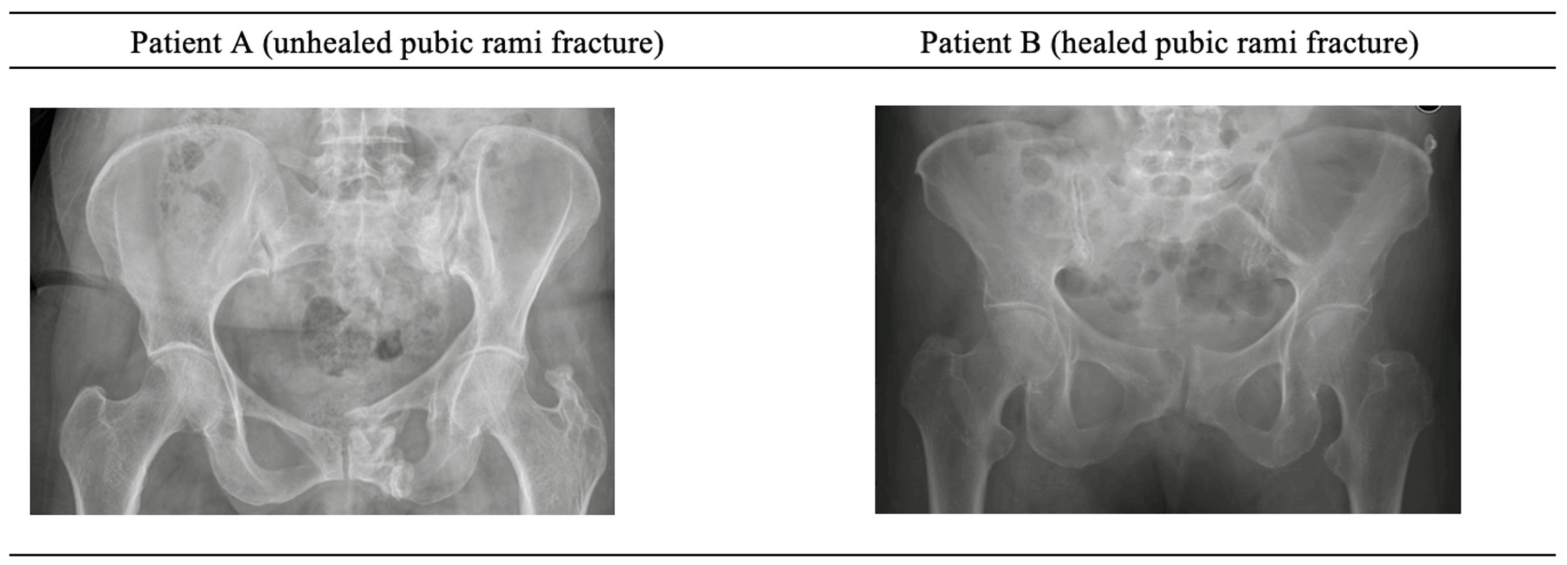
X-ray imaging at final follow-up for two patients in this cohort Patient A with an unhealed pubic rami fracture and Patient B with a healed pubic rami fracture
